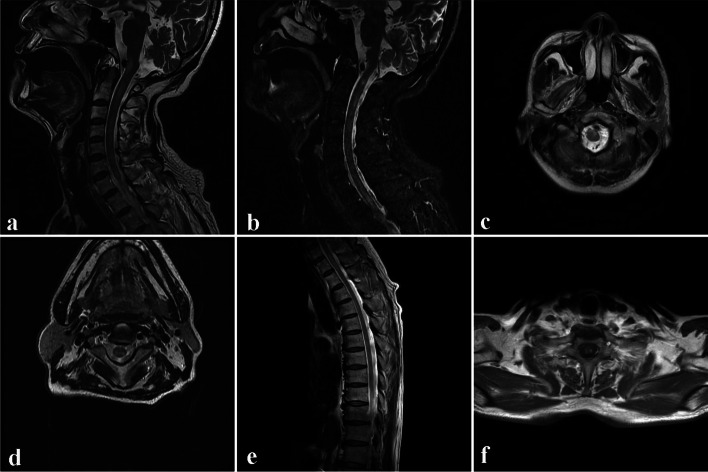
Magnetic resonance imaging of brain, cervical spine, and thoracic spine. Enhanced brian MRI showing uneven enhancement of medulla oblongata (a) and T2 hyperintensity lesions of medulla oblongata (b); Cervical spine MRI showing T2 hyperintense lesions in medulla oblongata and upper margin of the T2 vertebral body (c); A contrast-enhanced thoracic spine MRI showing uneven enhancement of T1 to T6 vertebral segments(d, e) and T2 hyperintense lesions of T1 to T6 vertebral segments (f)
